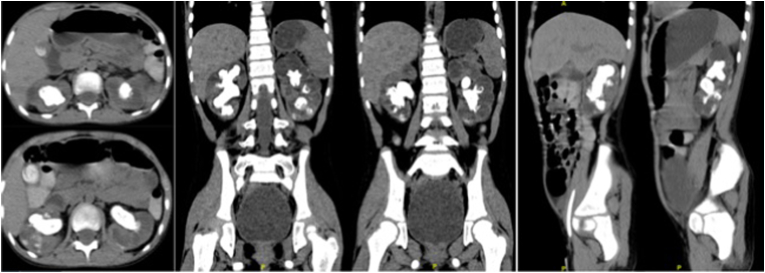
Non-contrast CT examination, showing bilateral staghorn nephrolithiasis.
